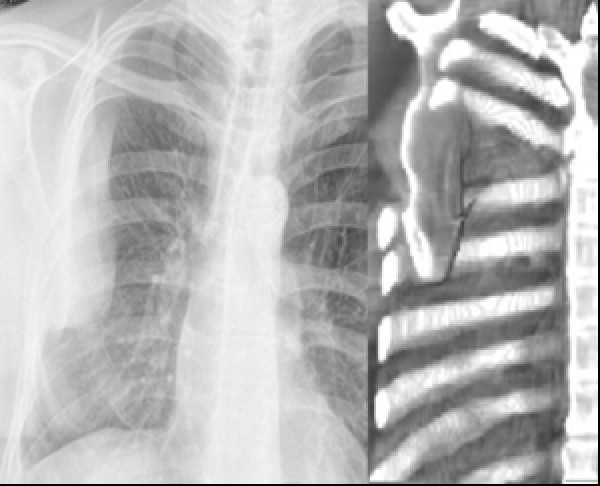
Computer reconstructions of CT scan of chest and chest x-ray showing internal dislocation of the scapula through the thoracotomy incision.
